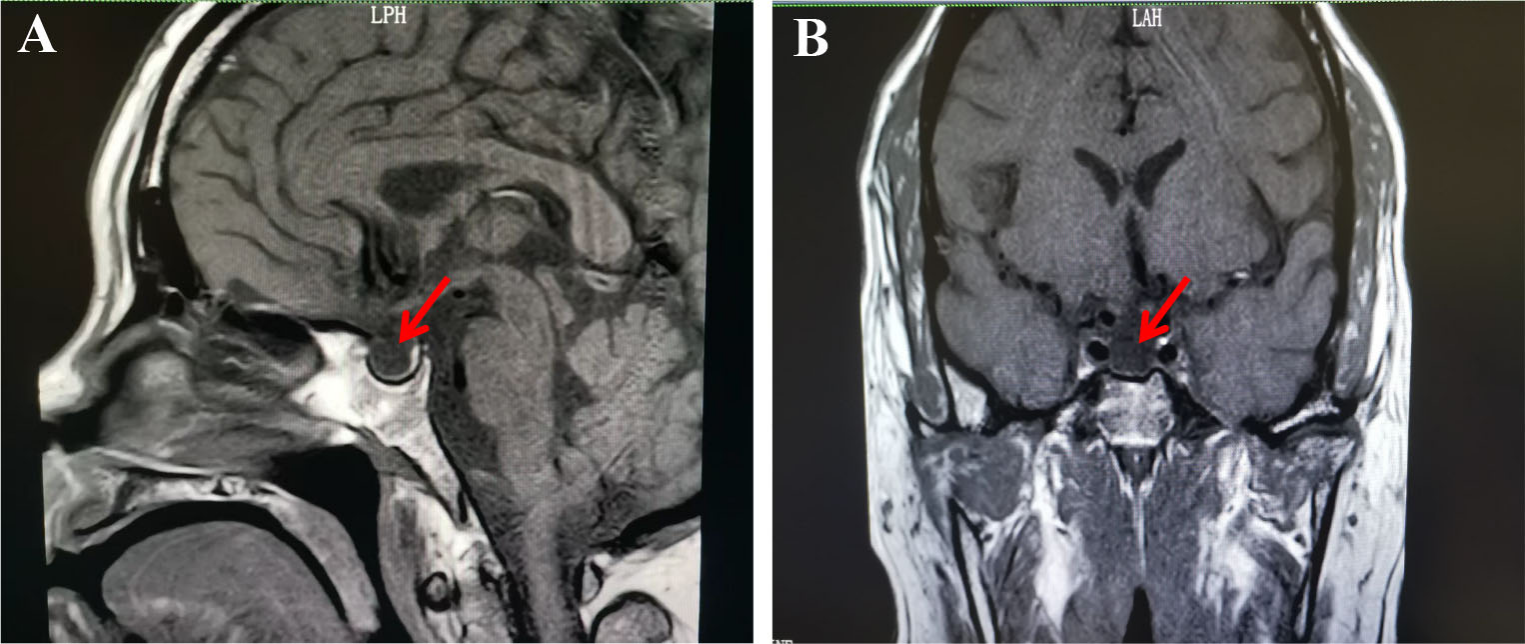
Enhanced pituitary magnetic resonance imaging shows empty sella.T1-weighted sagital (A) and coronal (B) magnetic resonance imaging reveal the sella filled with cerebrospinal fluid (red arrow).
